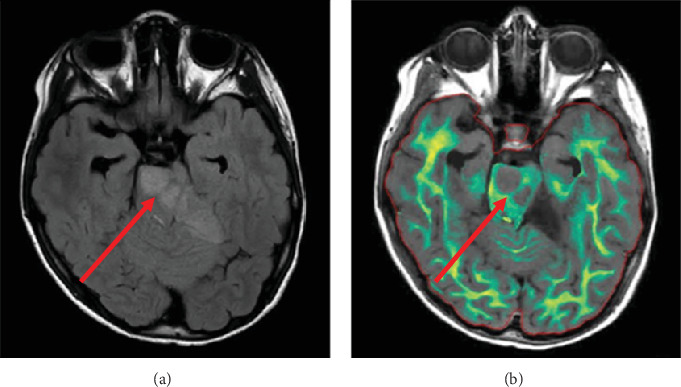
Brain MRI with myelin maps of high-grade glioma. Axial brain MRI demonstrating a high-grade diffuse midline glioma (red arrow) located in the pons on (a) FLAIR. (b) Myelin maps reveal an ill-defined, irregular margin of decreased demyelination.
